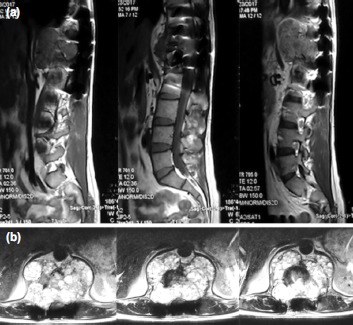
(a) Pre-operative MRI sagittal view (T1 Weighted - from left to right) showing the mass extending anteriorly involving T11,T12 and L1. (b) Pre-operative MRI axial view (T2 Weighted) at the level of T12 showing an expansile mass surrounding the T12 vertebrae.
